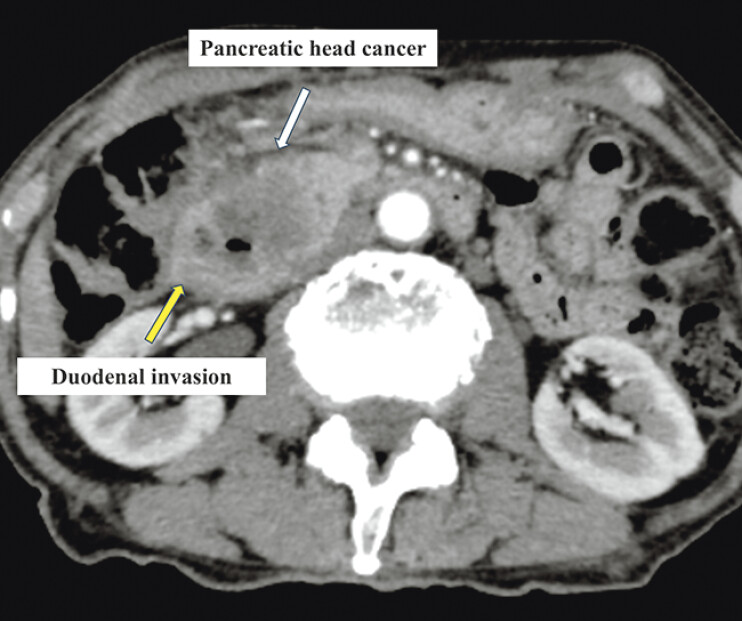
A computed tomography image demonstrating duodenal invasion caused by pancreatic head cancer.
